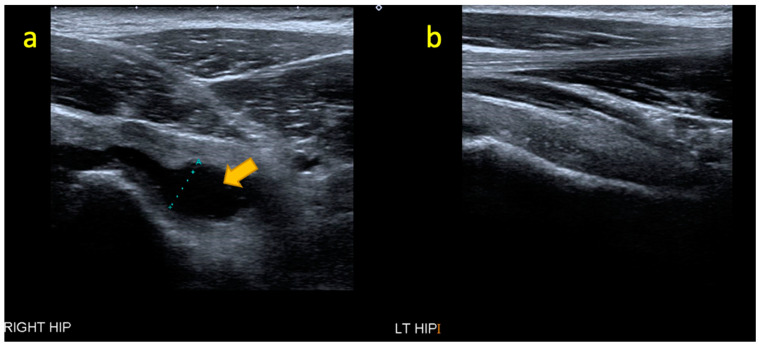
(a,b) Suspected septic arthritis of the right hip in a 4-year-old boy who subsequently had a surgical washout. Joint aspirate revealed CPPD crystals consistent with acute pseudogout. No pus cells in the aspirate and no organisms were grown. Ultrasound scan image of the right hip in the longitudinal plane shows a large joint effusion, containing a few echogenic debris in keeping with crystal deposition.
